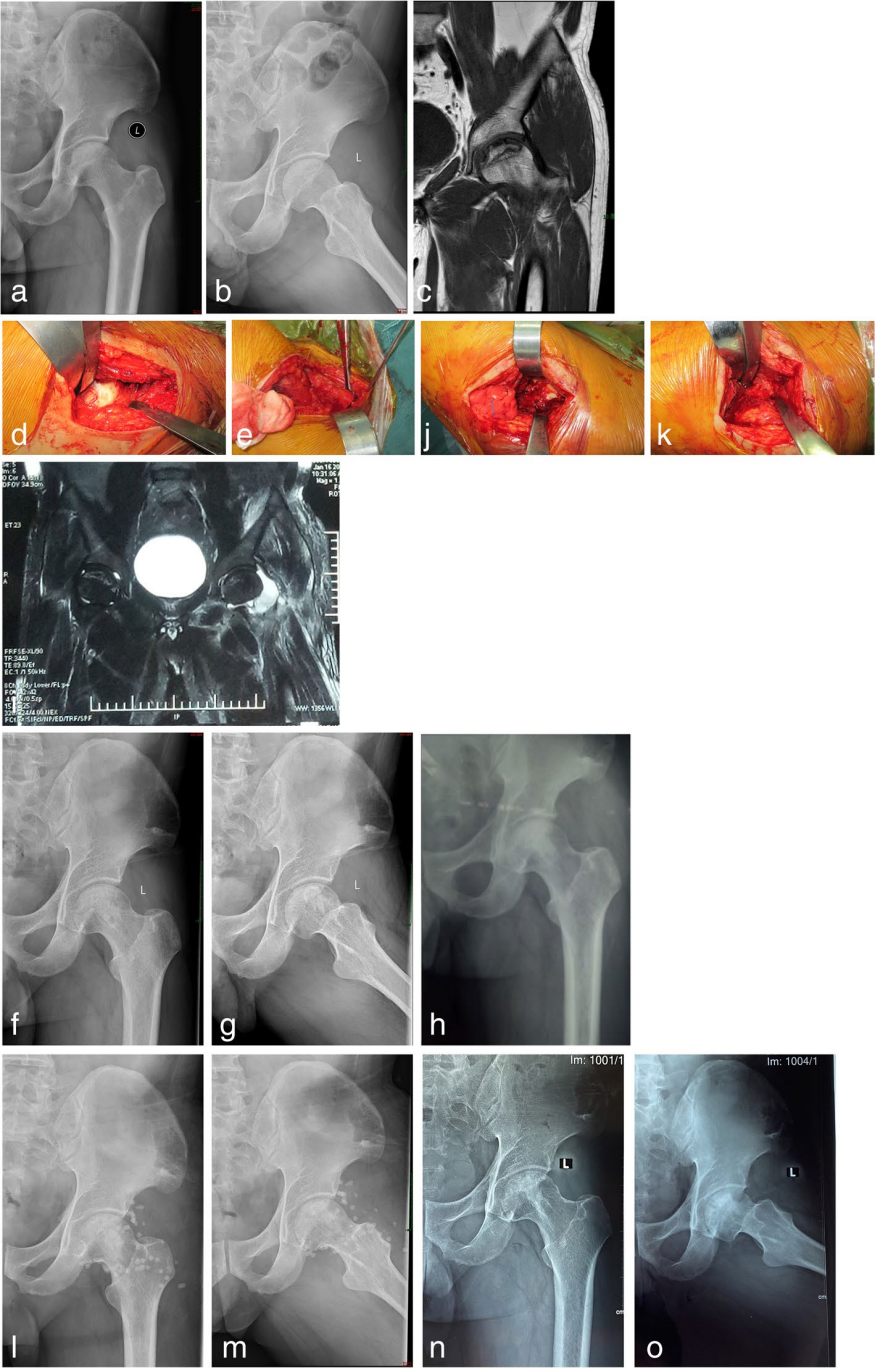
a, b Anteroposterior and frog-like radiographs of a 38-year-old male patient showing uneven density within the femoral head. c MRI T1WI showed femoral head necrosis. d OCM approach fenestration in the head and neck images of non-vascularized bone graft blocks occluding the bone window (black arrow). e Images of the ilium taken with subcutaneous tissue detached proximally from the same incision (black arrow). f, g Anteroposterior and frog radiographs of the hip at 3 months postoperatively showed increased bone density in the femoral head and good osteogenesis in the bone graft area. h At 6 months post-operation, the frontal radiograph of the hip joint showed good osteogenesis in the femoral head and swelling of the soft tissue around the hip due to high fever and pain in the operation area. i MRI T2WI showed synovial hyperplasia and edema in the joint. Edema of the muscle layer around the hip joint may indicate infection. j Original incision exposed the neck of the femoral head to visible intra-articular purulent secretions (black arrow). k Bone healing at the fenestration in the neck of the original femoral head (black arrow). l, m Anteroposterior and frog radiographs of the hip after debridement. n, o Anteroposterior and frog radiographs of the hip at the last follow-up show good osteogenesis of the bone graft region and stable femoral head
